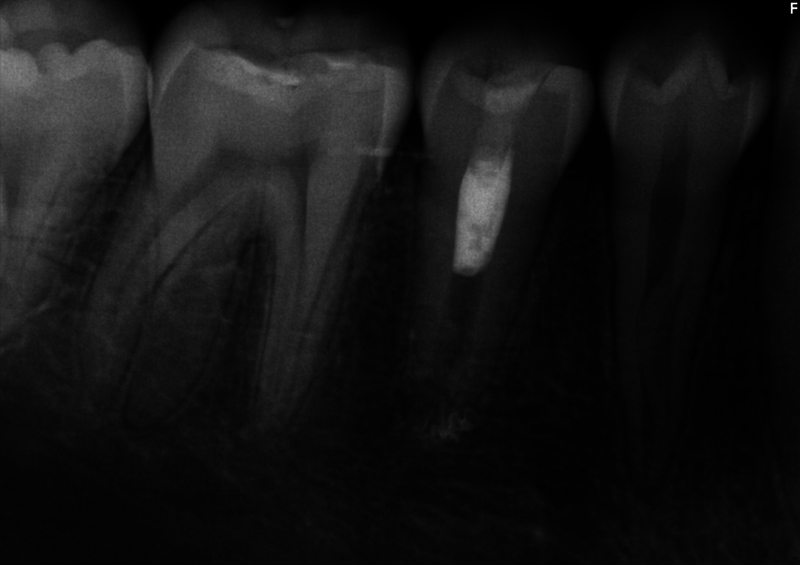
One-year follow-up periapical radiograph.
